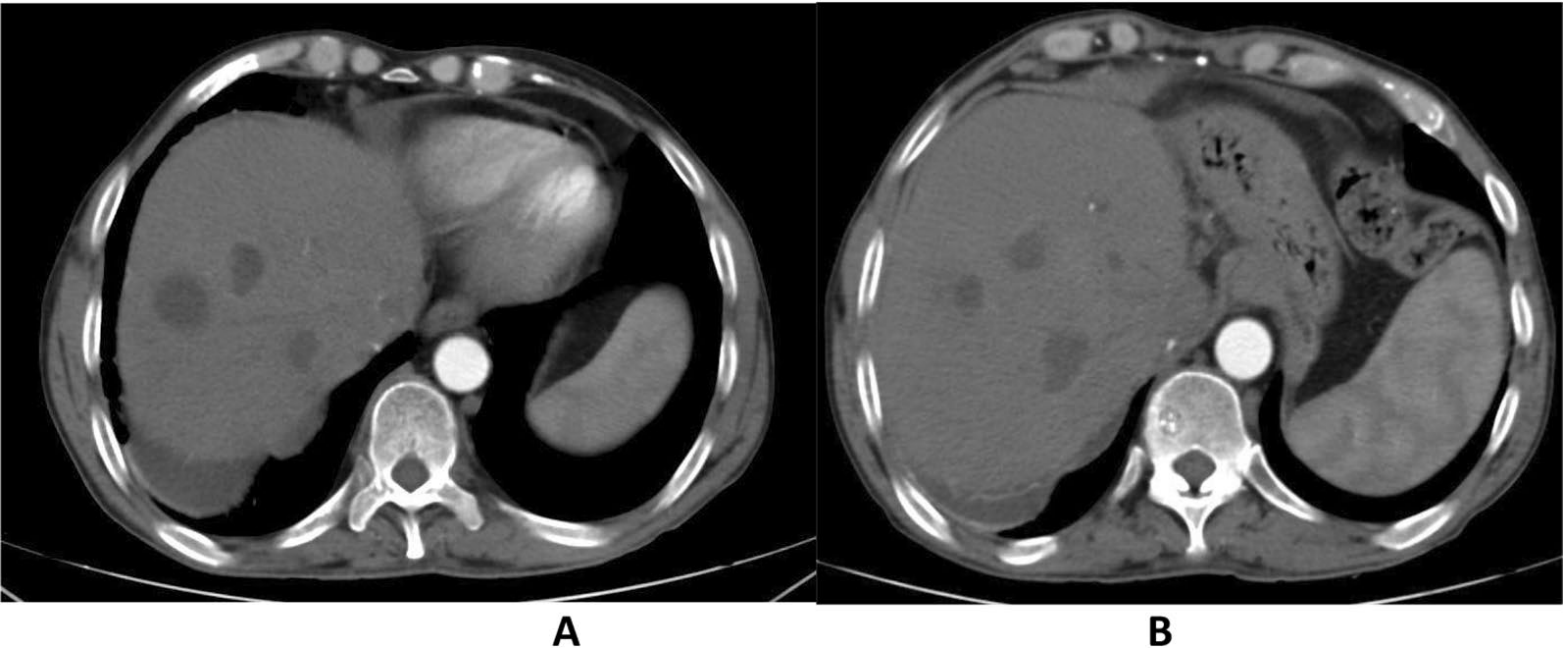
Contrast enhanced CT, arterial phase showing multiple hypodense cystic lesions with marginal enhancement denoting abscesses formation. (A) showing cholangectatic abscesses in segment VII, (B) showing cholangectatic abscesses in segments V & VI
